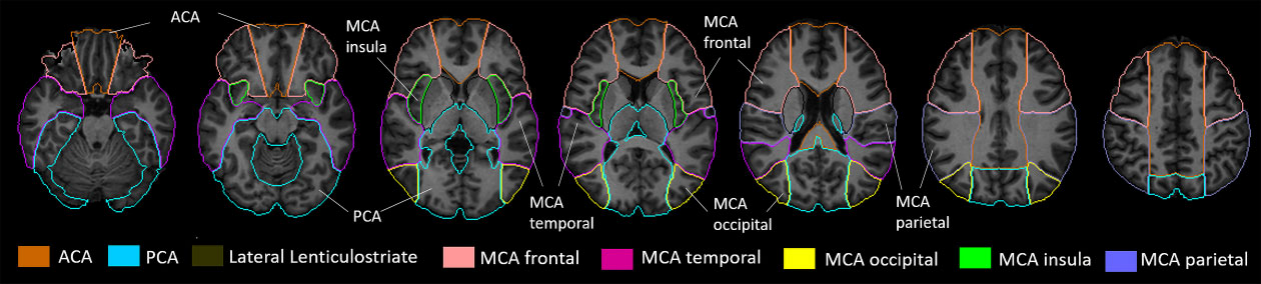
Digital three-dimensional brain MRI arterial territories atlas. This atlas is derived from Liu *et al*. (https://www.biorxiv.org/content/10.1101/2021.05.03.442478v2) Subdivisions of biological importance in the MCA territory were based on probabilistic maps and/or anatomical landmarks. ACA and PCA territories were not separated, as they were less commonly affected territories.
